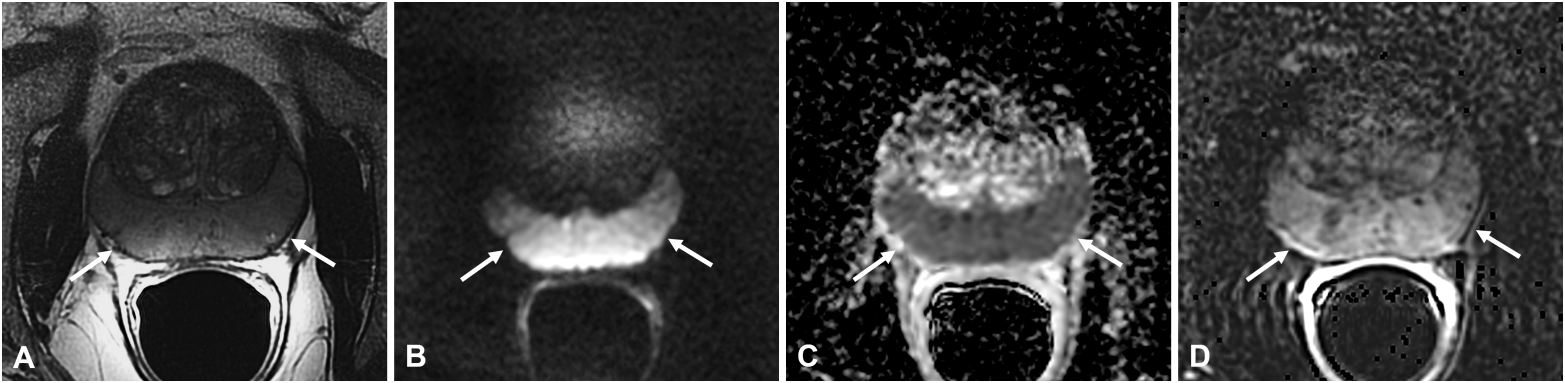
Non-specific granulomatous prostatitis in a 66-year-old patient with a PSA value of 15.92 ng/ml. The axial T2-weighted sequence shows diffuse hypointensity and thickening of the peripheral zone (PZ) with preservation of the glandular capsule and pseudocapsule (arrows in A). The entire PZ appears diffusely hyperintense on the DWI image (arrows in B) and markedly hypointense on the ADC map (arrows in C) with diffuse high contrast enhancement on the DCE image (arrow in D).
